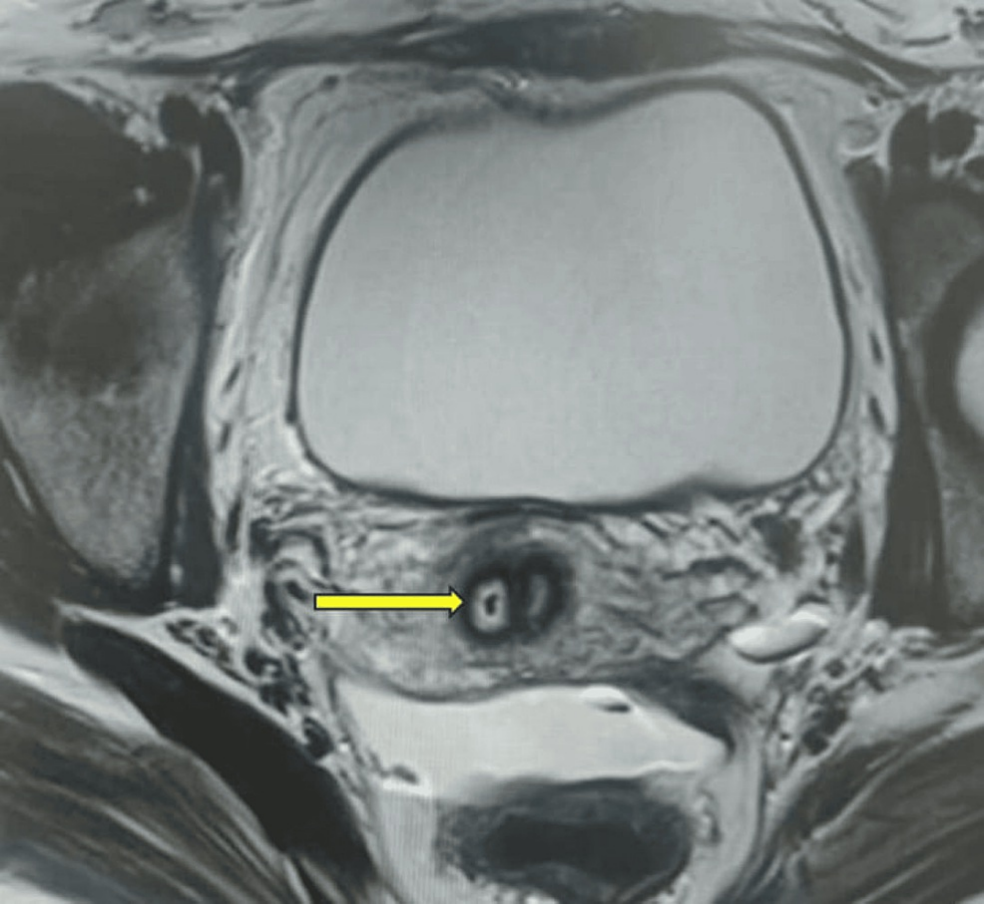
Transverse MRI section depicting a double cervix (yellow arrow points at the owl eye appearance of the double cervix on MRI)
